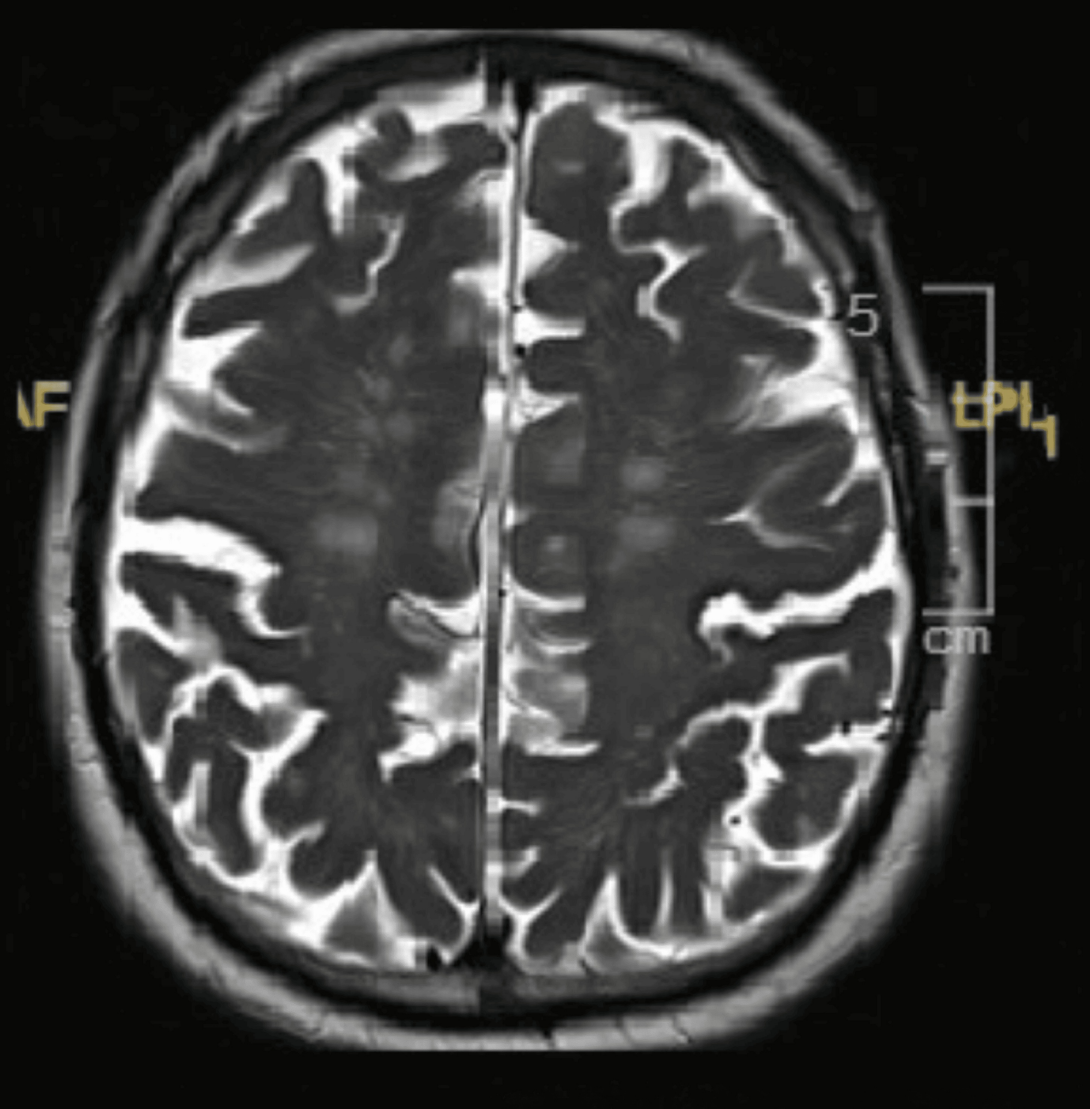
T2-weighted images showed mild diffuse cortical atrophy and small blood vessel ischemic changes.
